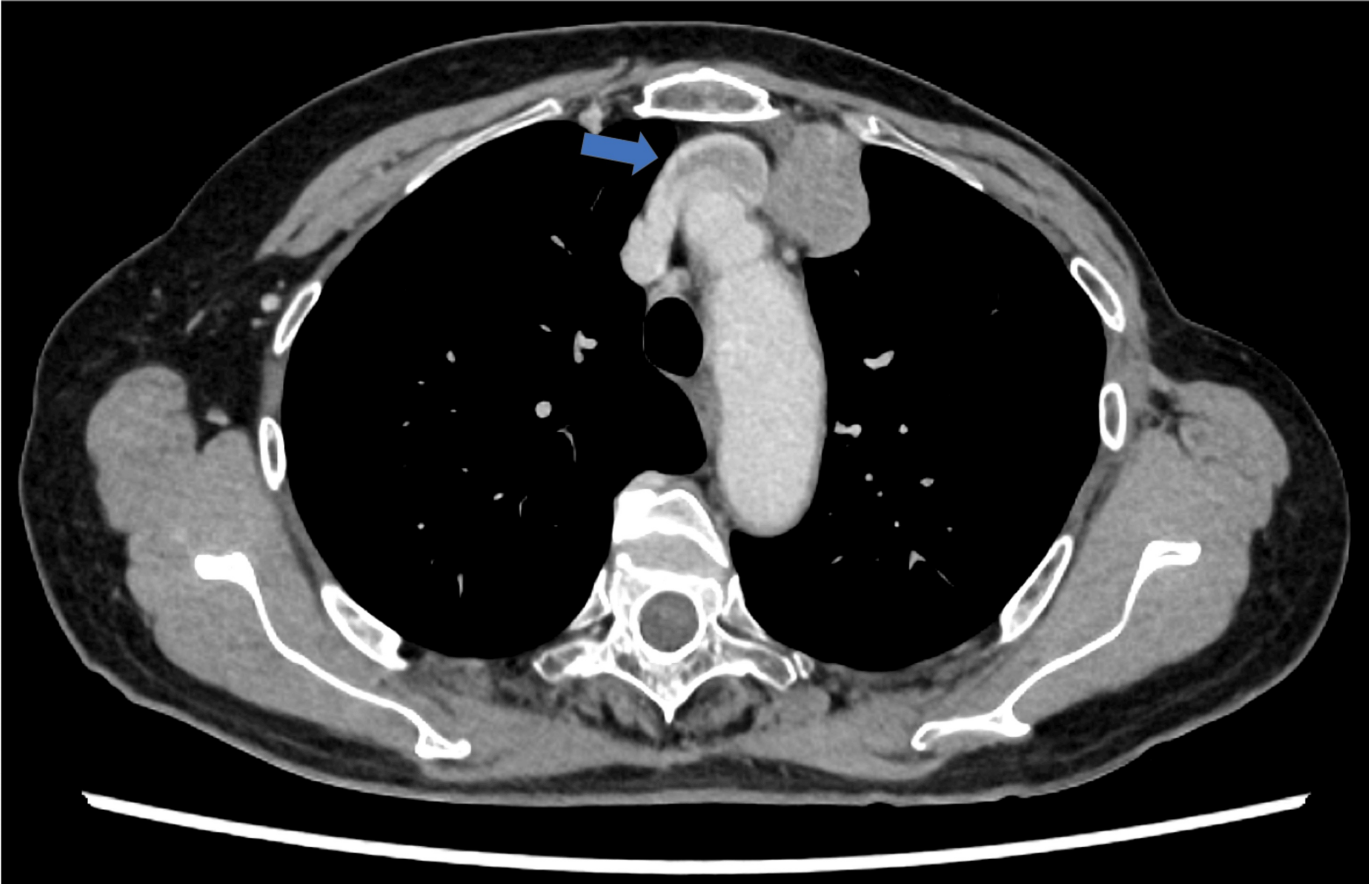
Tumor thrombus extending into the SVC on axial CT A tumor thrombus extending from the left brachiocephalic vein to the SVC (blue arrow). The PET-CT showed FDG accumulation in the tumor thrombus. SVC: superior vena cava; FDG: fluorodeoxyglucose
